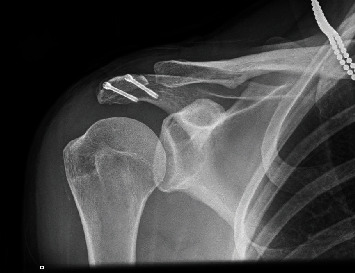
Postoperative radiograph of the final position of the screws.
